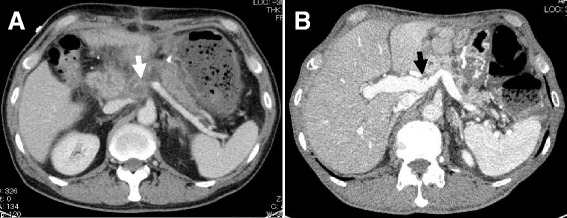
A comparison of the portal vein stenosis assessed by CT images of the portal phase between right before (a) and 10 months after (b) stent graft placement. Portal vein stenosis (a, *white arrow*) caused by postoperative pancreatic fistula was significantly improved (b, *black arrow*)
